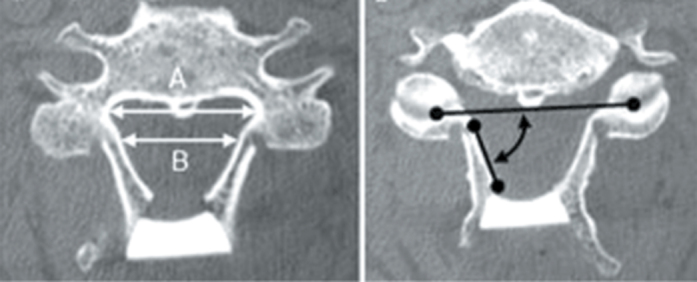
Bony spinal parameters measured on axial computed tomographic images. Left: Gutter position. (A) Transverse diameter of the spinal canal (B) Distance between bilateral gutters. Right: lamina inclination angle.
